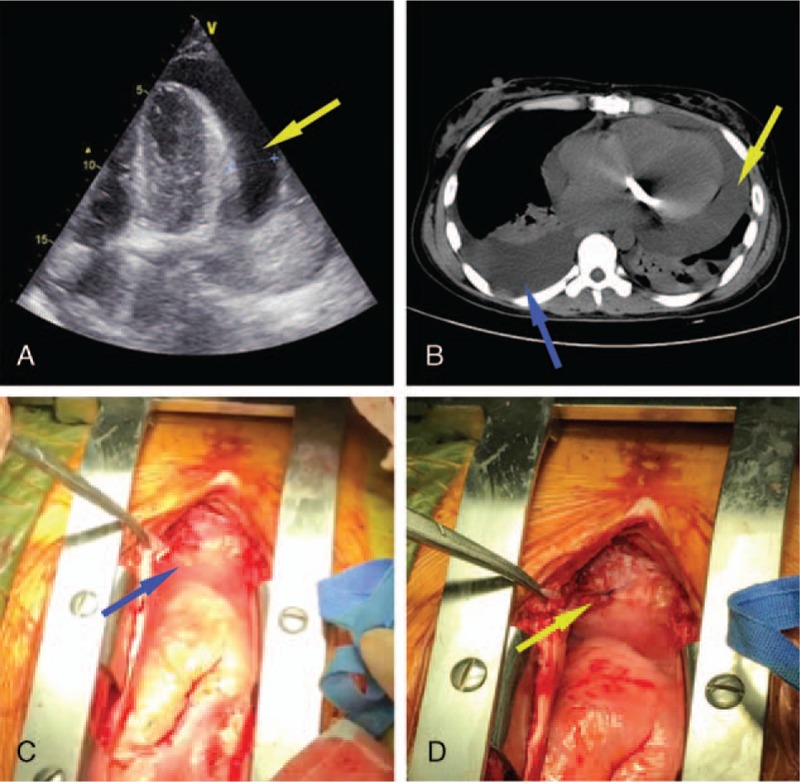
(A) Echocardiography showed moderate-to-massive pericardial effusion (yellow arrow). (B) Computed tomography showed massive pericardial effusion (yellow arrow), pleural diffusion on the right side and partial pulmonary atelectasis (blue arrow). (C) An opening of a duct, with light yellow fluid (blue arrow) flowing out continuously, on the diaphragm. (D) The visualized superior phrenic lymphatic fistula was closed with a continuous suture (yellow arrow).
